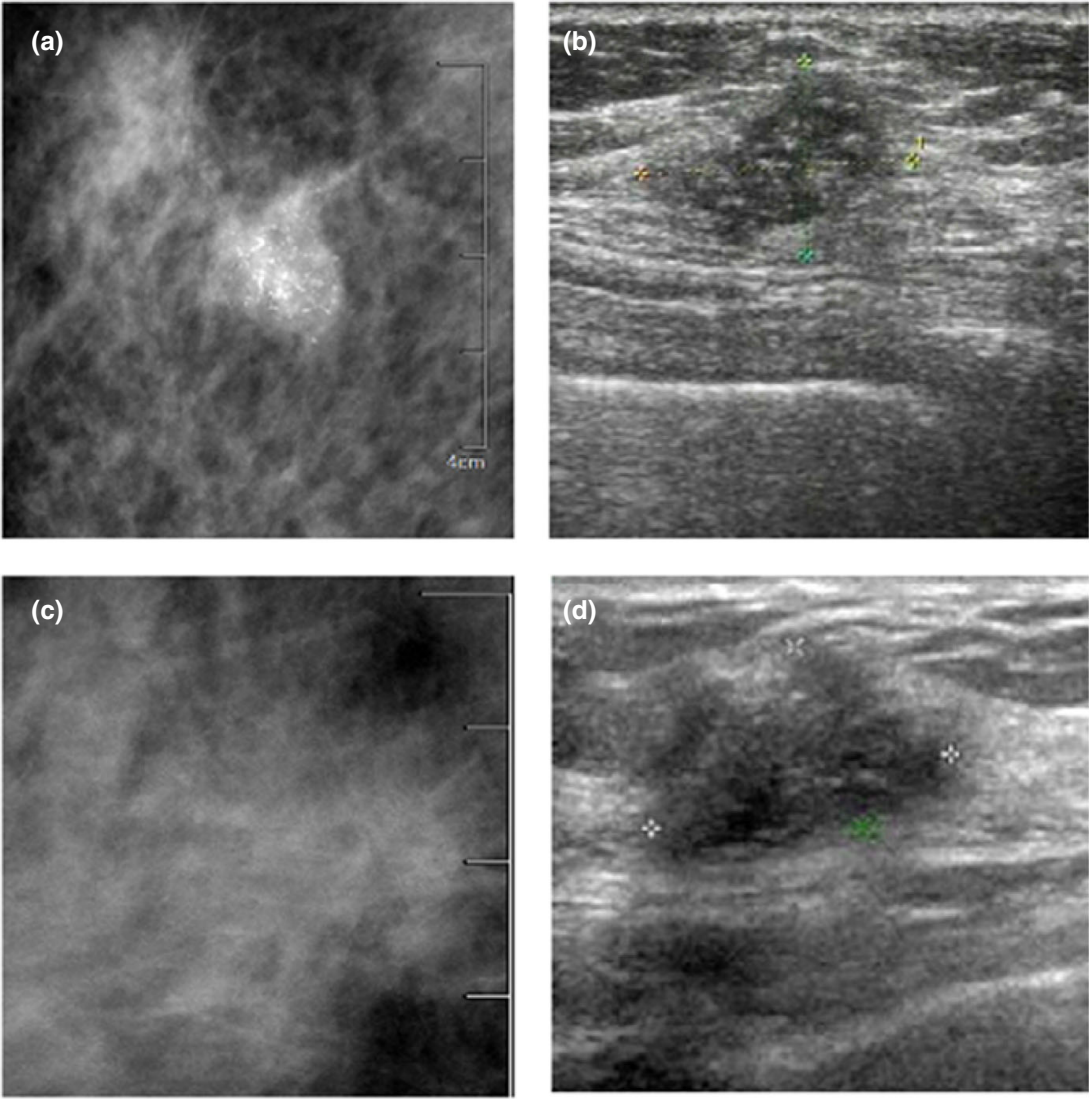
(a) A 57‐yr‐old ductal carcinoma in situ with microinvasion (DCISM) female patient. A1, mammography detects the presence of round high‐density node with a clear border and multiple calcifications. A2, Ultrasound shows a hypoechoic mass with an unclear border and multiple hyperechoic spots.(b), A 45‐yr‐old DCISM female patient. B1, mammography reveals a solid mass with an unclear border and irregular morphology. B2, Ultrasound shows the hypoechoic irregular mass with an unclear border.
